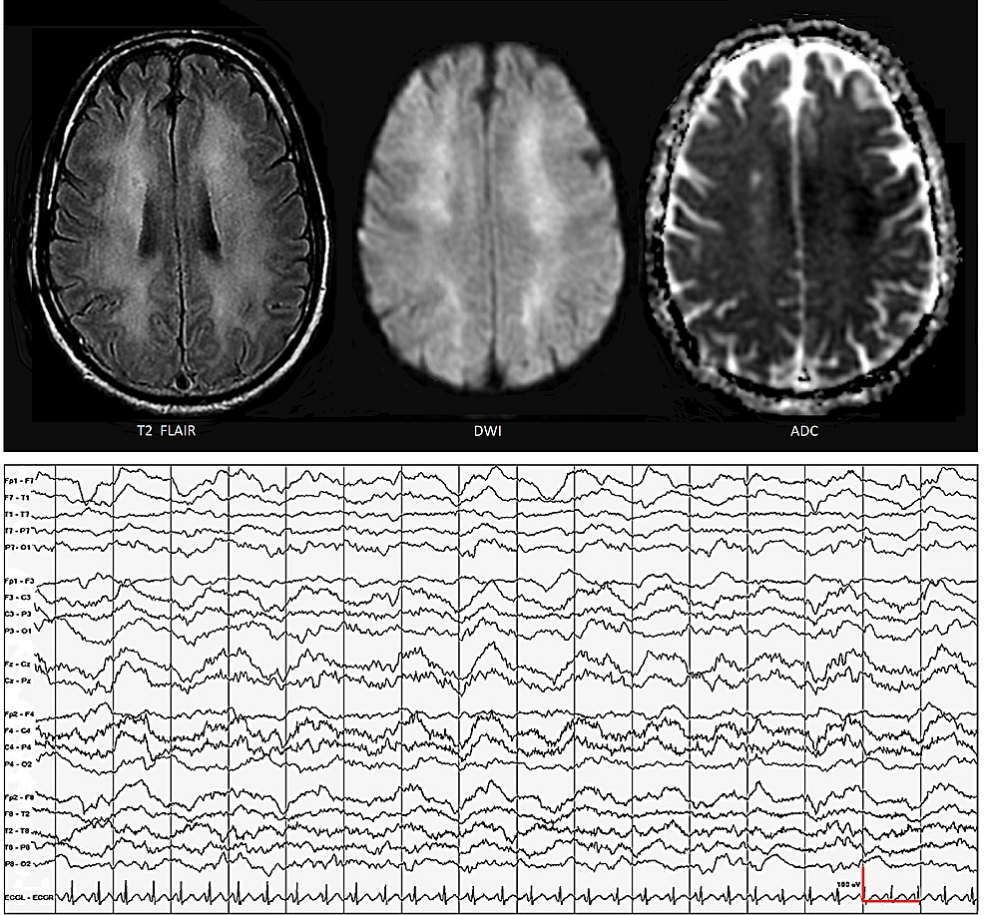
During readmission for abulia and psychomotor retardation, brain MRI showed extensive bilaterally symmetric deep white matter hyperintensity with patchy areas of restricted diffusion and EEG showed diffuse polymorphic delta activity indicating widespread demyelination or axonal injury in the white matter.
